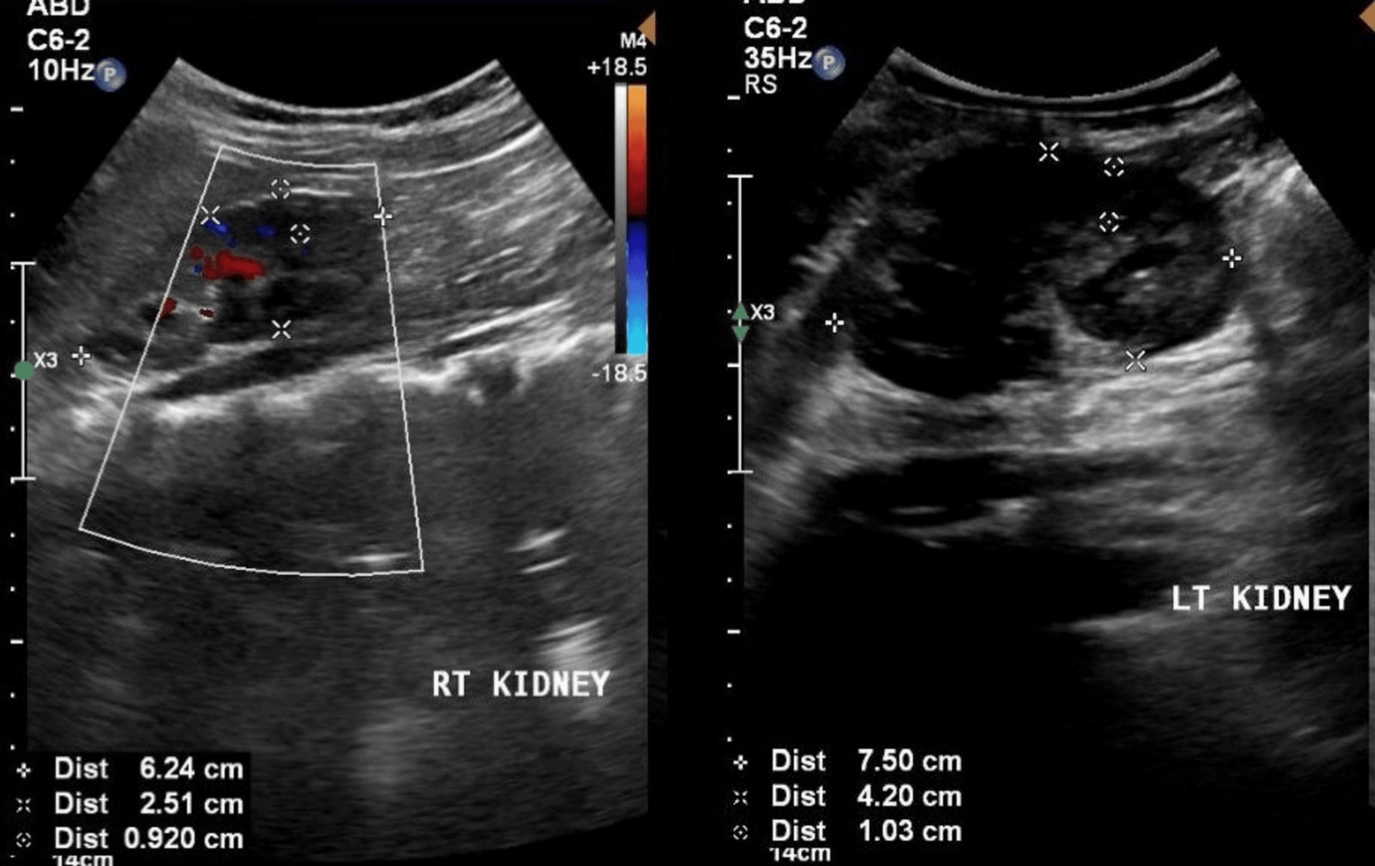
Ultrasound exam of the kidneys, ureters, and bladder (US-KUB) demonstrated improvement in backpressure changes, although mild hydronephrosis persisted.
